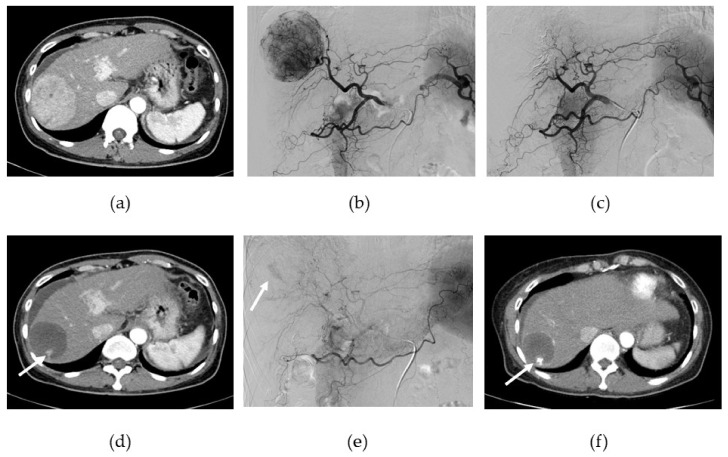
A 53-year-old woman who underwent DEB-TACE and one subsequent session of Cis-TACE for a single HCC 7.2 cm in size. (a) Contrast-enhanced axial computed tomography (CT) image in the arterial phase before initial DEB-TACE, showing an arterial enhancing mass in the right hemiliver. (b) Common hepatic arteriography of the patient during initial DEB-TACE, showing a hypervascular tumor in the right hemiliver. (c) Angiogram after selective embolization of the tumor-feeding arteries with HepaSpheres, showing complete devascularization of the tumor in the right hemiliver. (d) Enhanced axial CT image 5 weeks after DEB-TACE, showing PR with small residual arterial enhancement (arrow). (e) Common hepatic arteriography during subsequent Cis-TACE, showing a small enhancing lesion (arrow) in the center of the necrotic tumor. The tumor-feeding arteries were selectively embolized (not shown). (f) Enhanced axial CT image 4 weeks after subsequent Cis-TACE, showing no demonstrably enhancing portion in the liver and treated tumor, with lipiodol accumulating solely in the viable portion (arrow).
